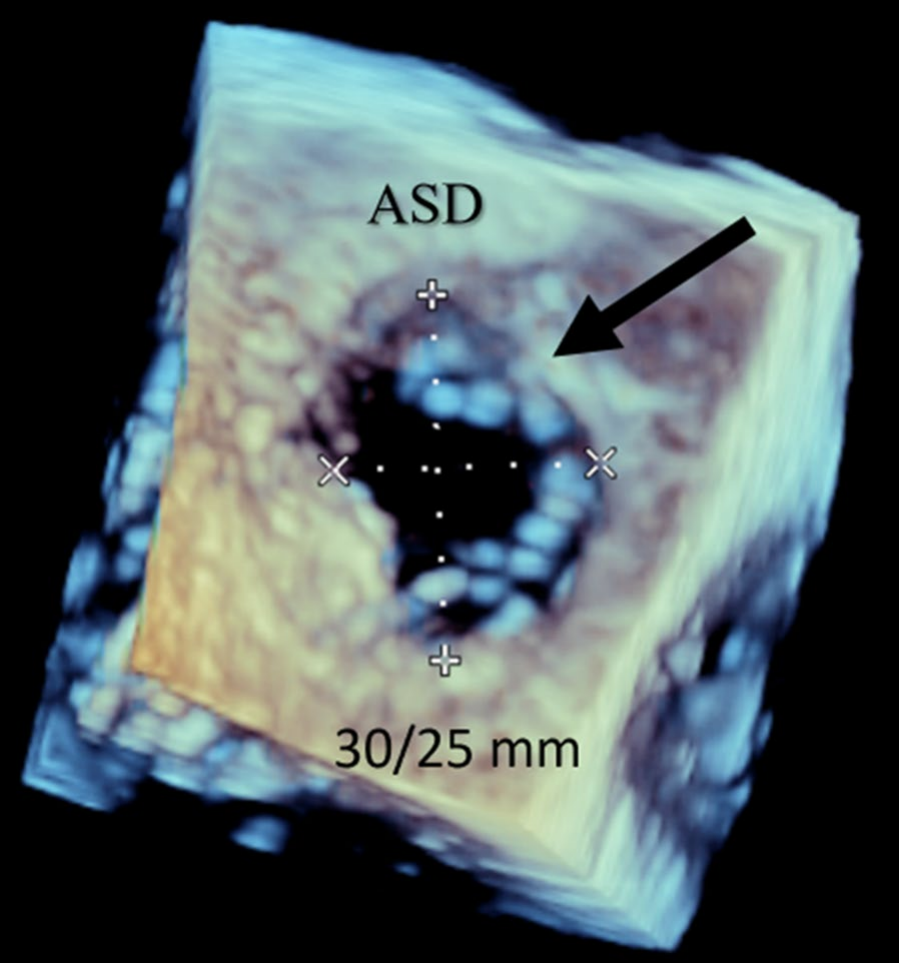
3D imaging of the secundum ASD on TEE. 3D imaging of the ASD on TEE has confirmed a secundum ASD (black arrow) with left-to-right shunt, maximum end-to-end diameter of 30/25 mm, area +/− 4.2 cm^2^, and adequate rims size (12 mm superior vena cava rim, 16 mm inferior vena cava rim, 11 mm atrioventricular valve rim, < 5 mm aortic rim). *3D* three-dimensional, *ASD* atrial septal defect, *TEE* trans-esophageal echocardiography
